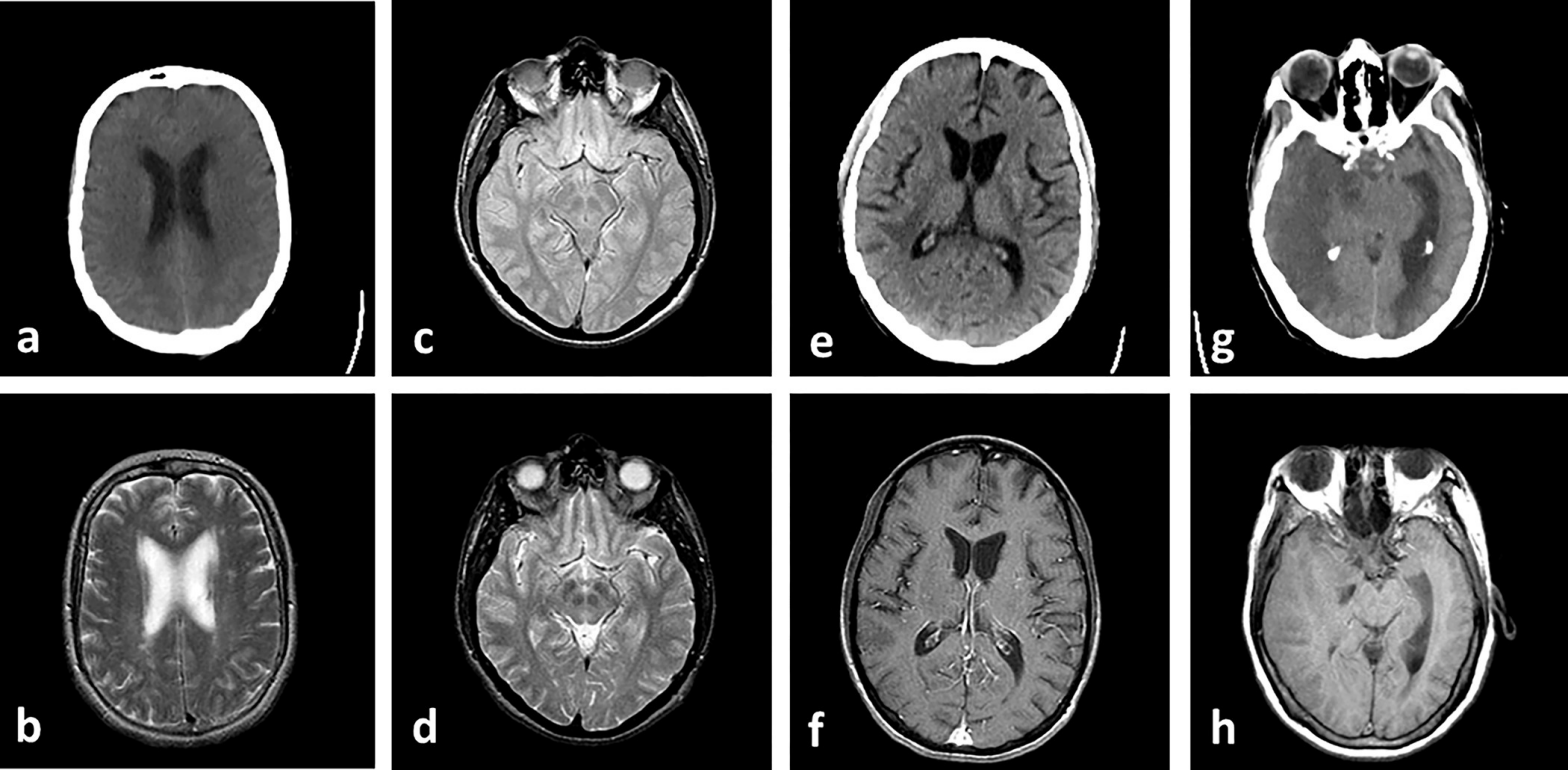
Experiment 9–12: Fusion of Multimodal medical images of patients with vascular dementia. (a, b) Experiment 5: CT and MR-T2 images. (c, d) Experiment 6: MR-PD and MR-T2 images. (e, f) Experiment 7: CT and MR-GAD images. (g, h) Experiment 8: CT and MR-T1 images.
